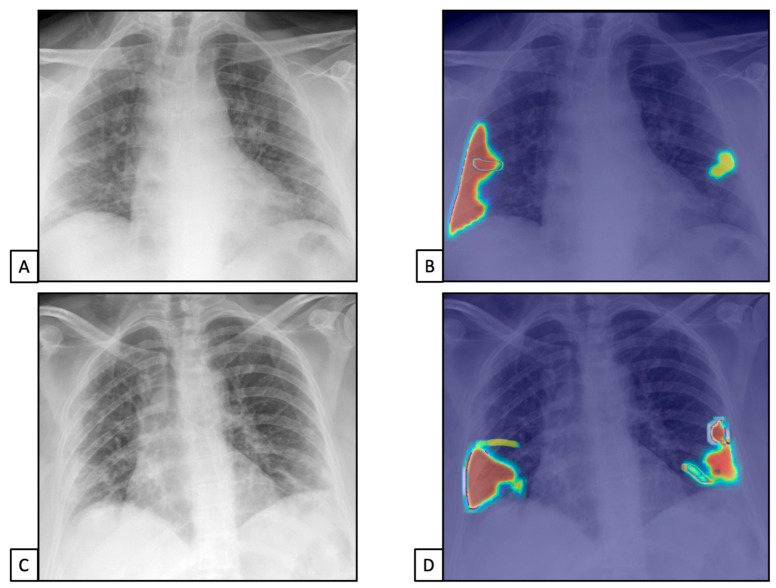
Chest X-rays of patients admitted to the emergency department with the suspicion of COVID-19 infection belonged to dataset 1. (A,C) represent CXRs acquired at the bedside, showing multiple slight interstitial and alveolar opacities located peripherally and with a lower distribution. (B,D) The AI system analysis obtained in a few seconds displays the pathological zones. The AI system reported a high suspicion of COVID-19 infection (99.99%). The final diagnosis was lung involvement by COVID-19 pneumonia.
